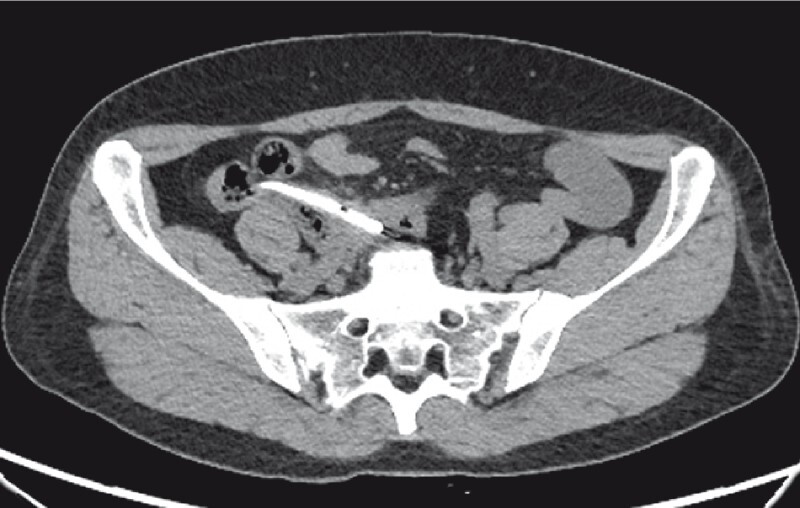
Follow-up CT after 2 months showed that the periappendiceal abscess had fully disappeared and the stent was in place.
